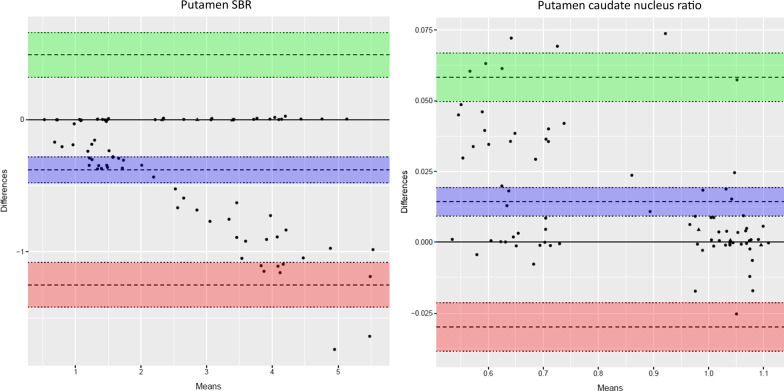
Short versus longer acquisition protocol. Bland–Altman plots of 17–42-min reconstructions versus 30–40-min reconstructions for same patients as in Fig. 3. Triangles represent patients with discrepancy between PET and SPECT reading. The purple area represent the mean difference with 95% confidence limits (C.I.), the green area represent the upper limit of agreement with 95% C.I., and the red area the lower limit of agreement. Please note the relative higher values for putamen SBR (left) using the shorter reconstruction with 95% of patients being within 25% difference. Putamen/caudate nucleus ratio (right) show similarly relatively higher values but the difference is smaller and within 10%. The difference stress the need for using the same acquisition protocol in a future normal database for comparison
